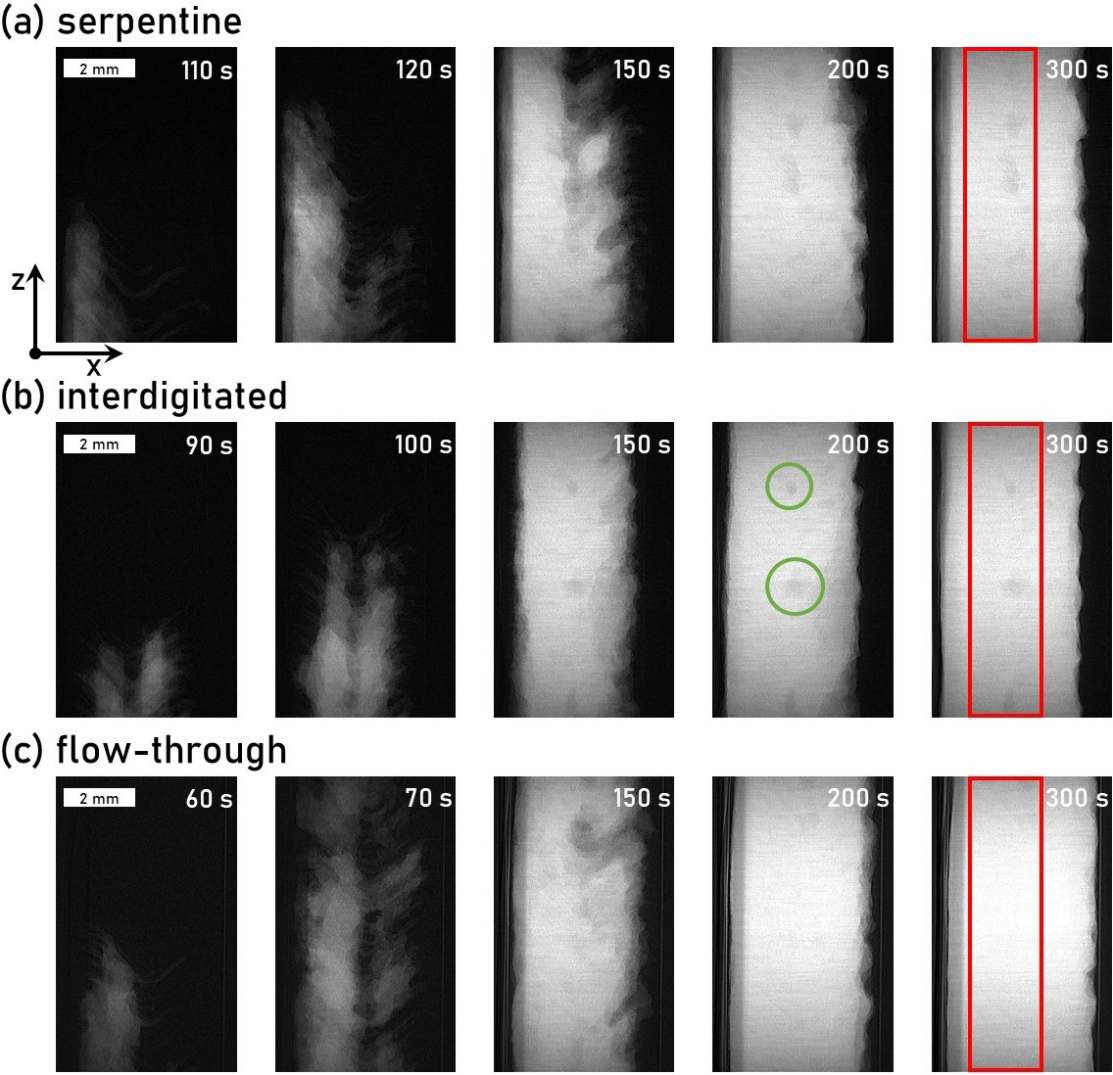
Visualization of the vanadium electrolyte at specific times during its injection by the means of X‐ray radiography through (a) serpentine and (b) interdigitated flow fields, as well as (c) a flow‐through configuration. The brighter the image is, the higher the electrolyte thickness at this point. Darker spots signify the presence of trapped air bubbles inside the porous electrodes; two examples are marked with a green circle in (b). The red box in each last image shows the region of interest, which was selected to calculate the average saturation.
